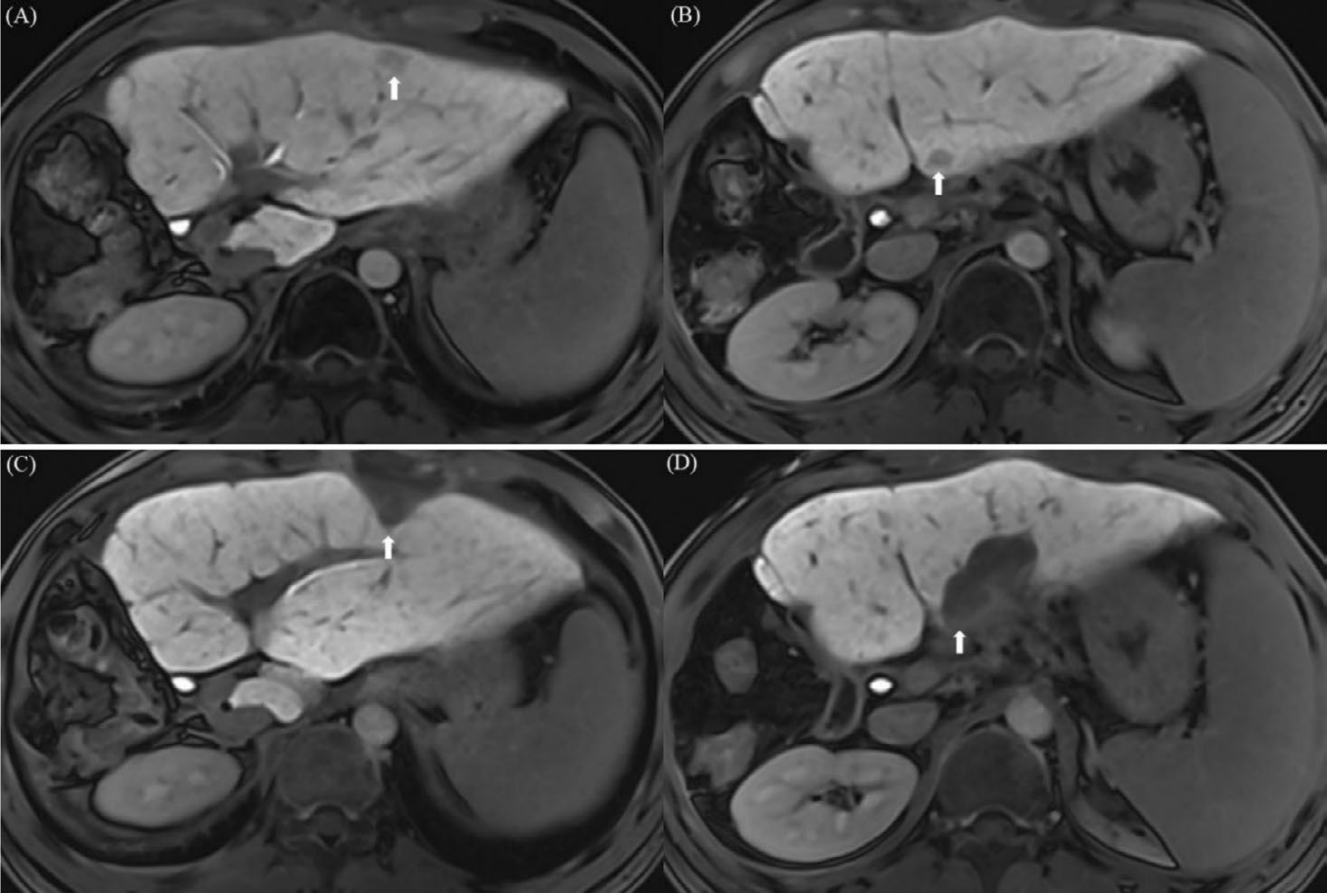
(A and B) Gd‐EOB‐DTPA‐enhanced MRI scans reveal two de novo nodules (white arrow) in the left hepatic lobe. (C and D) After MWA, imaging examinations reveal the complete ablation of both nodules (white arrow).
